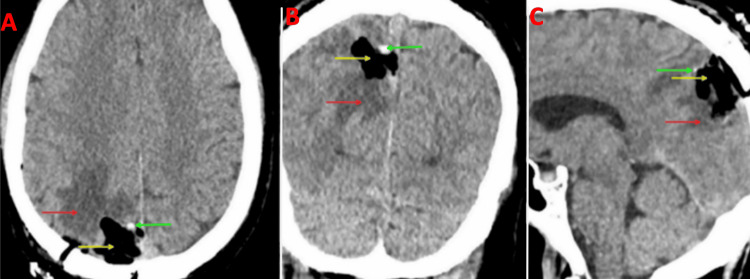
Postoperative CT head with views of the axial (A), coronal (B), and sagittal (C) planes demonstrating interval postoperative changes of right parietal-occipital craniotomy for resection of previously visualized mass Red arrows: Persistent vasogenic edema in the right parietal-occipital lobes, Yellow arrows: Postoperative pneumocephalus, Green arrows: Tiny amount of blood in the operative bed No mass, hemorrhage, or hydrocephalus are seen. No major vessel vascular territory infarct is seen. No intra or extra-axial fluid collection is seen.
